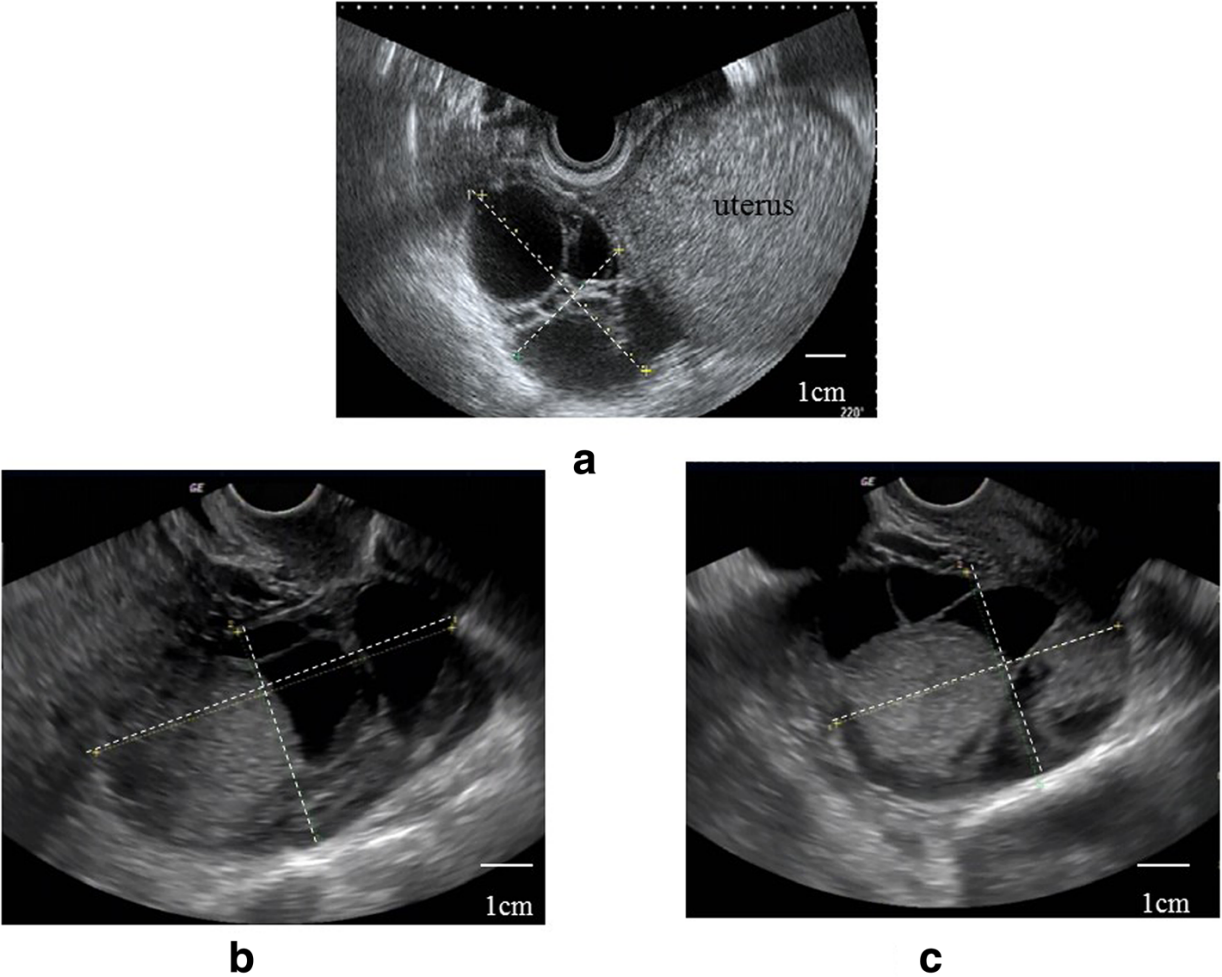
Transvaginal ultrasonograms of the ovarian cysts. a A 71 × 43–mm right ovarian cyst on postoperative day 4. b A 82 × 43–mm right ovarian cyst on postoperative day 7. c A 66 × 50–mm left ovarian cyst on postoperative day 7
